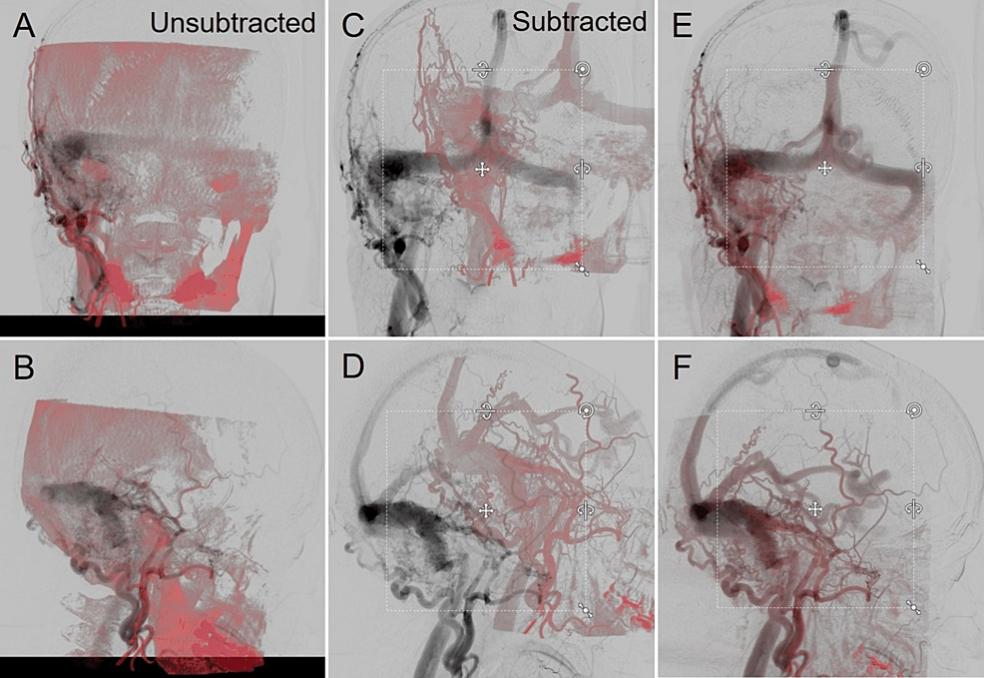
Comparison of Unsubtracted and Subtracted CBCTA on the Results of Co-Registration with 2D-DSA for Right TS-DVAF. A, B: unsubtracted CBCTA; C–F: subtracted CBCTA (C, D: pre-fusion; E, F: post-fusion). Note the difference in the selectivity of the vasculature images from each CBCTA.
